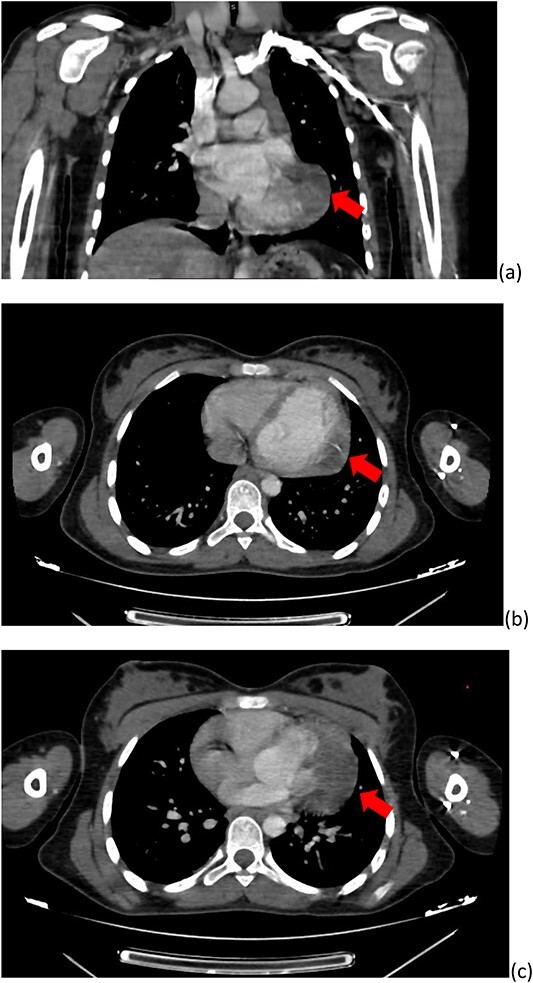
Axial (a) (b) and coronal (c) thoracic computed tomography with contrast revealing hypodense cystic lesion on left ventricular myocardium (arrow) not enhancing after contrast injection.
